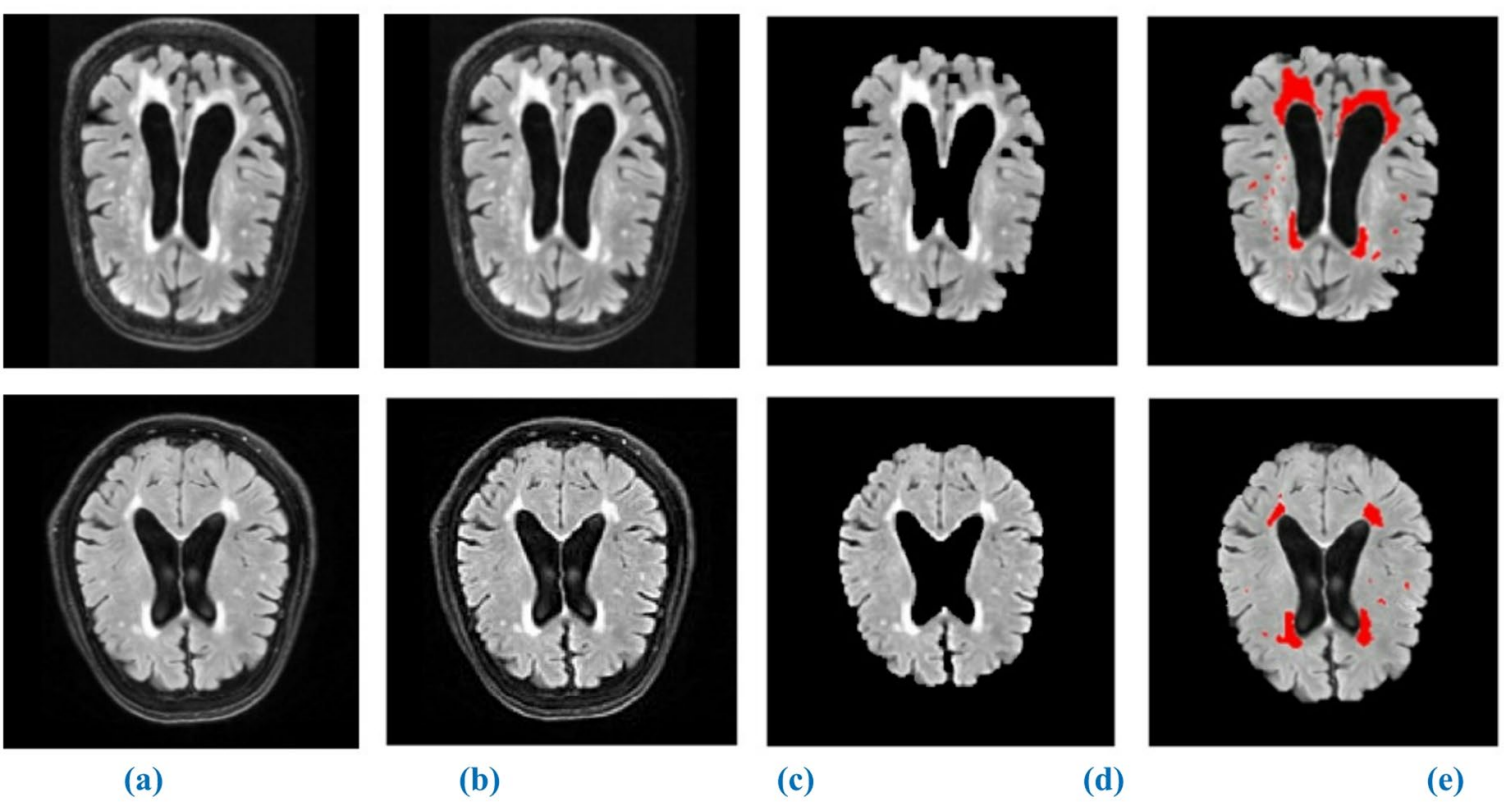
Segmentation of WMH on high-resolution T1-w MRI images. (a) original image, (b) enhanced image (c) skull stripping, (d) binary mask image, (e) Segmented output.
